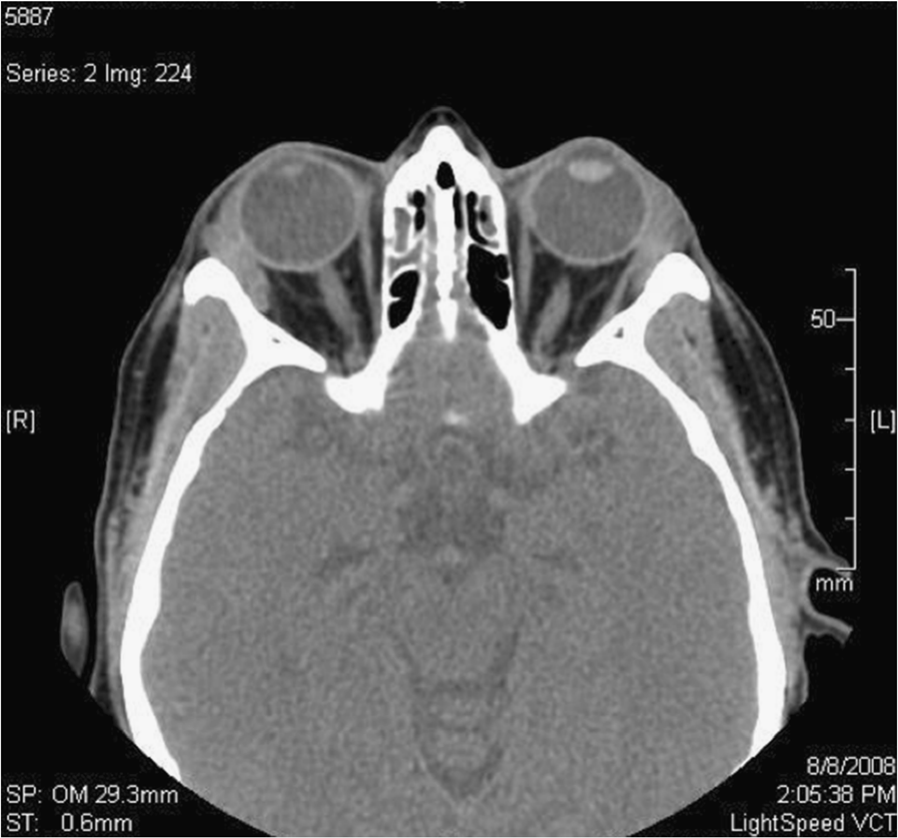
High resolution computed tomography of the orbits showing bilateral lacrimal gland enlargement.
